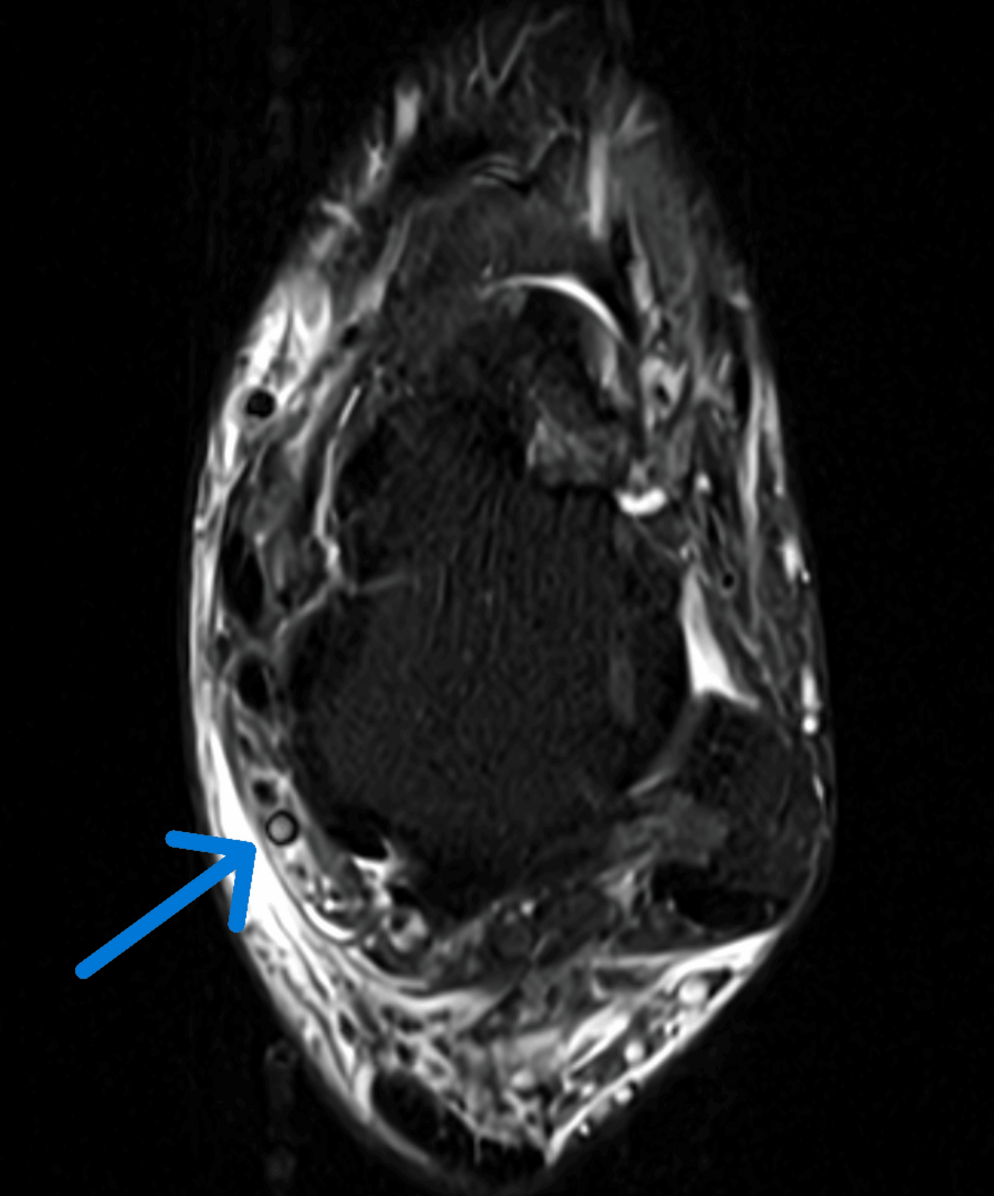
MRI revealing vein thrombosis near the ankle Vein thrombosis medial to the talus.
